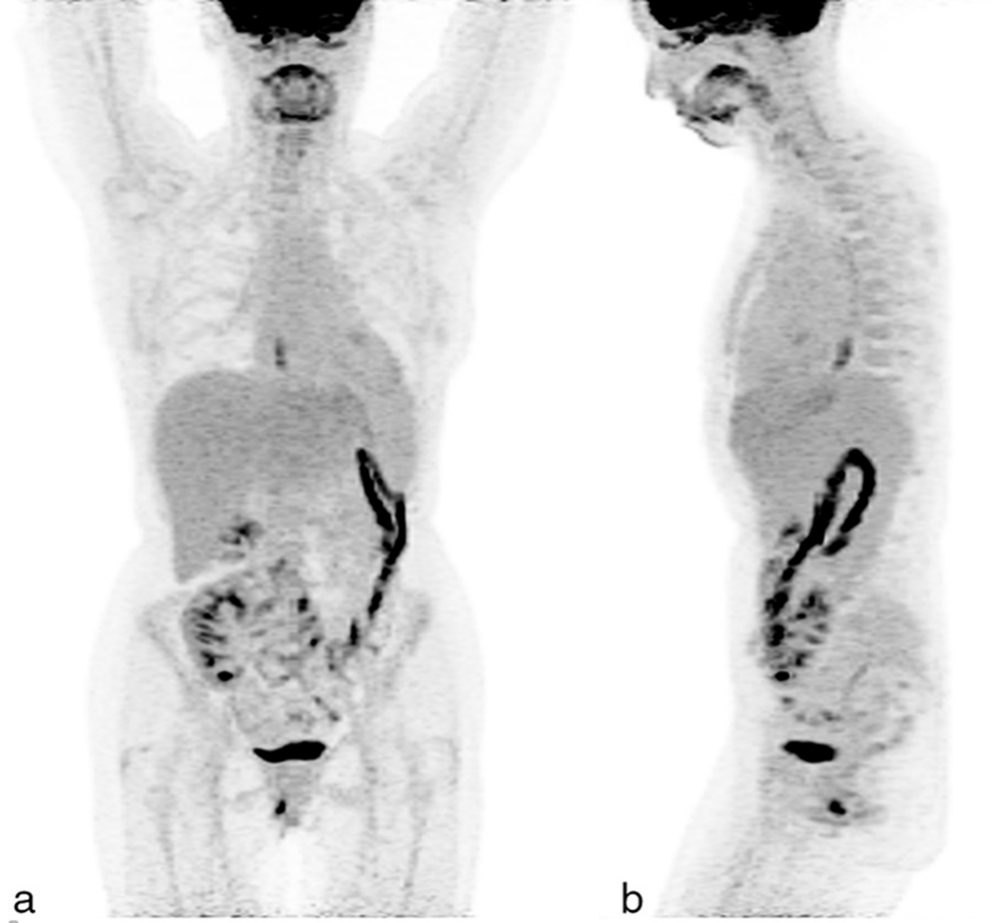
A follow-up ^18^F-fludeoxyglucose positron emission tomography/CT scan performed 12 months after the completion of rituximab therapy. (a) Anterior and (b) lateral maximum intensity projection images do not show any ^18^F-fludeoxyglucose-avid lung lesions, confirming disease remission.
